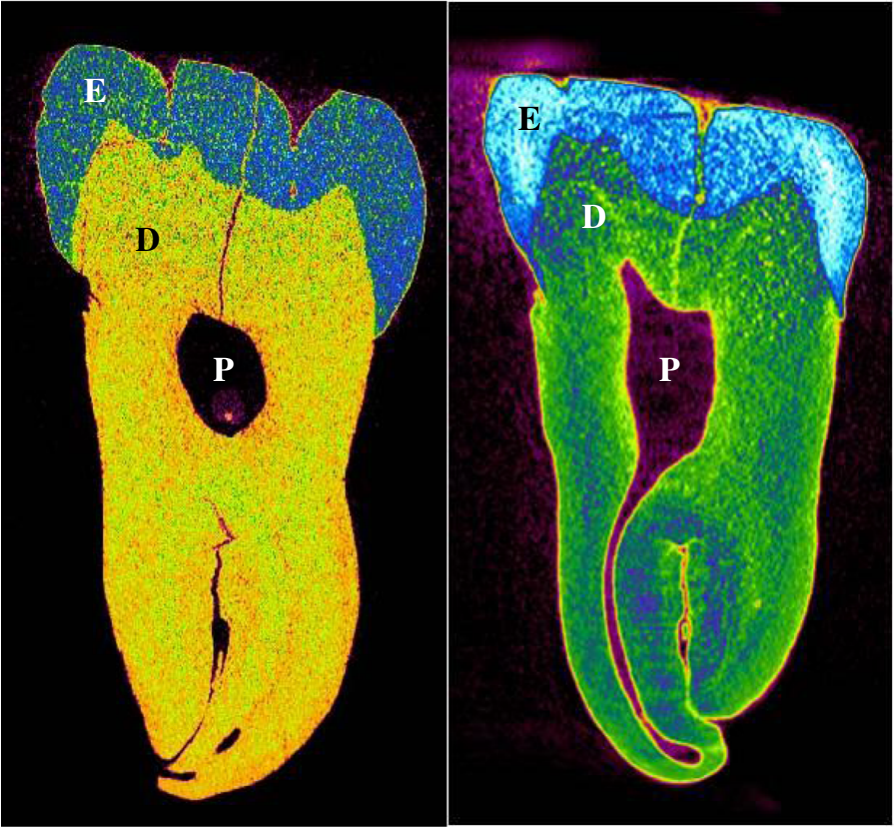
μ-CT scans of two different teeth showing all three major layers including the pulp cavity.*E*, enamel; *D*, dentine; *P*, pulp.
